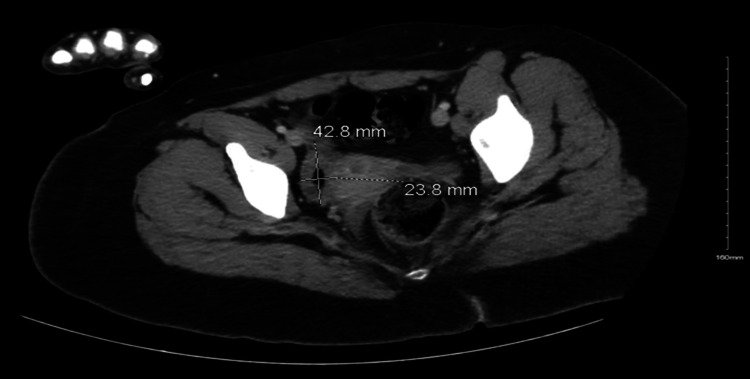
CT scan of the pelvis demonstrating a 42.8 mm by 23.8 mm right ovarian teratoma.
